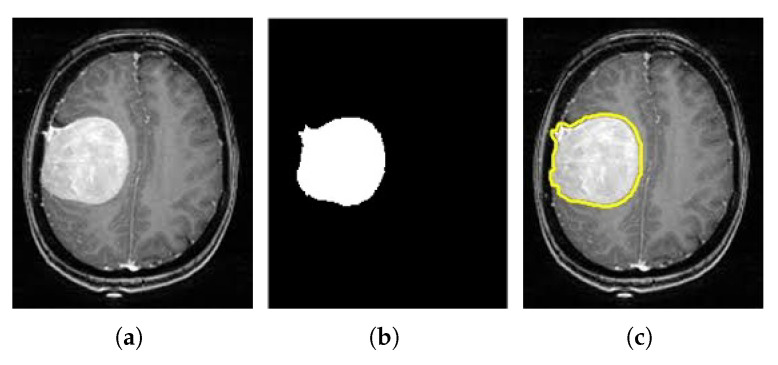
Meningioma brain image. (a) Source MRI, (b) Segmented tumor image, and (c) Extraction of tumor.
